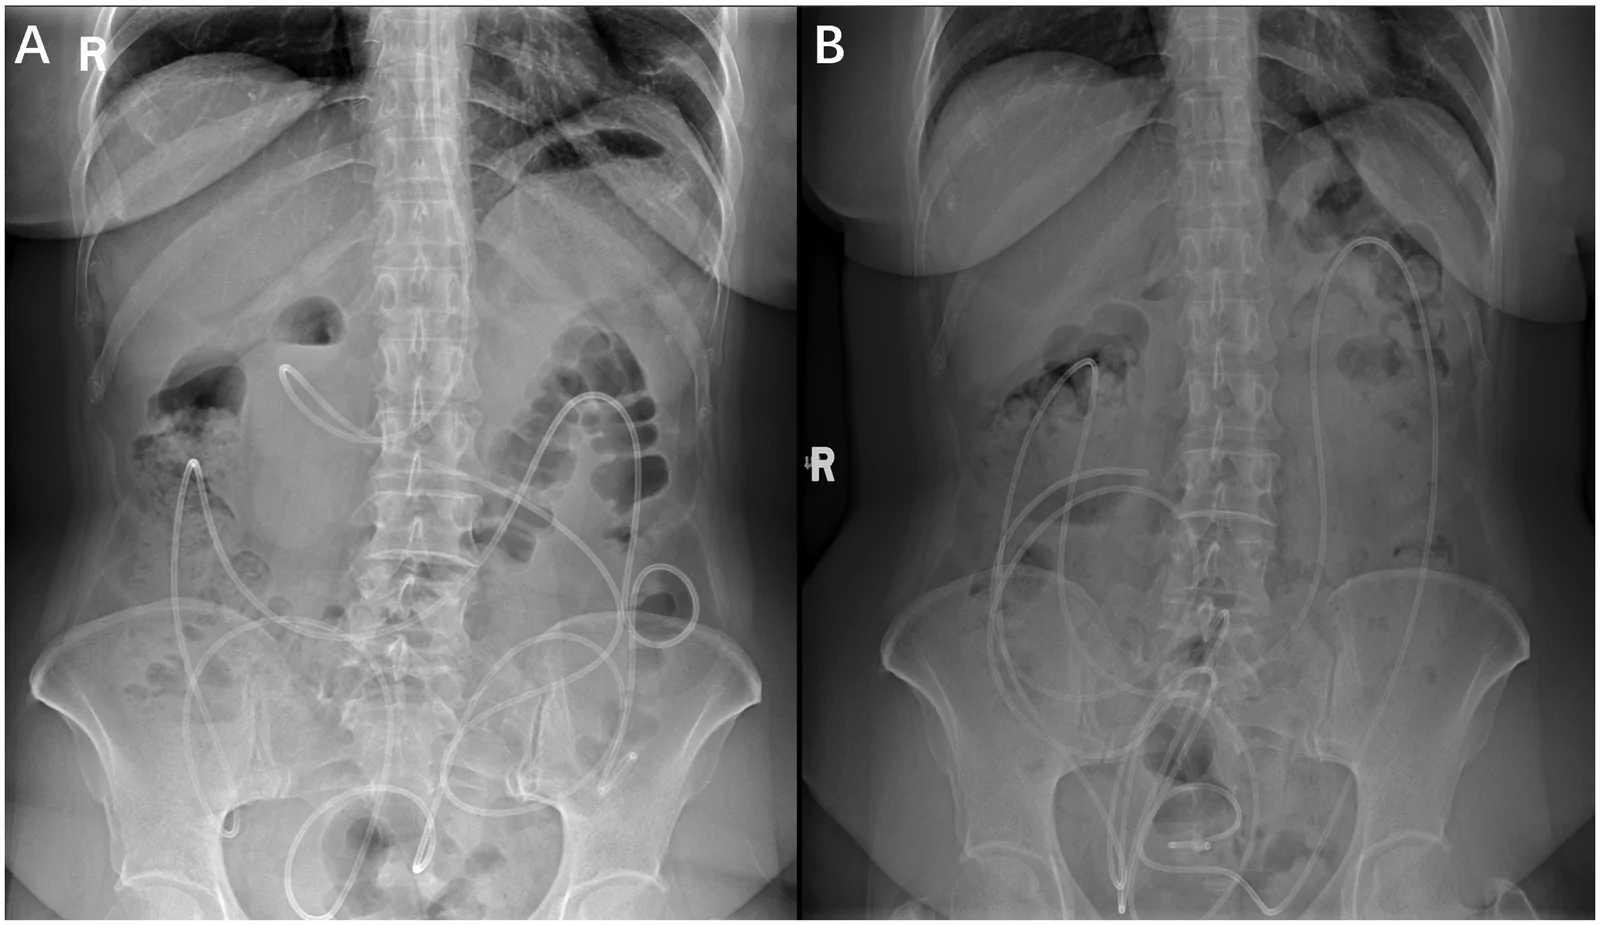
(A) Radiography revealed a tubular shadow in the region of the esophagus, stomach, and intestines, with the distal end located within the intestinal loops in the left lower abdomen. (B) Radiography showed a tubular shadow in the abdominal intestinal region, with the proximal end located within the pelvic cavity.
